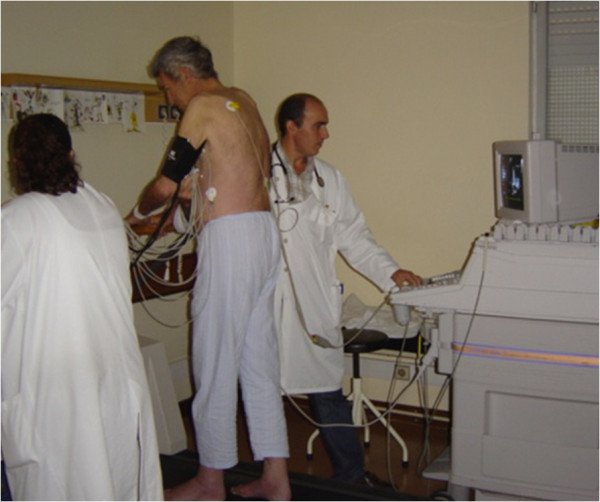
Echocardiographic data acquisition with the patient in orthostatic position during exercise in treadmill.
